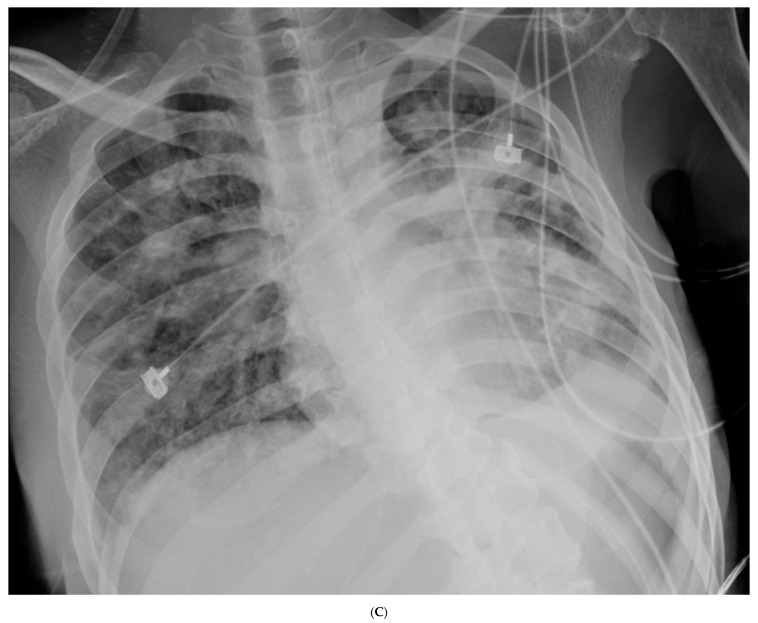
Chest radiograph on ICU Day 1 showing bilateral diffuse infiltrates. (A) On ICU Day 0, at admission, showing diffuse bilateral alveolar infiltrates consistent with severe ARDS. (B) On ICU Day 10, after prone positioning and anti-TB therapy initiation, showing persistent infiltrates but some improvement in aeration. (C) On ICU Day 50, prior to ICU discharge, showing substantial resolution of infiltrates.
